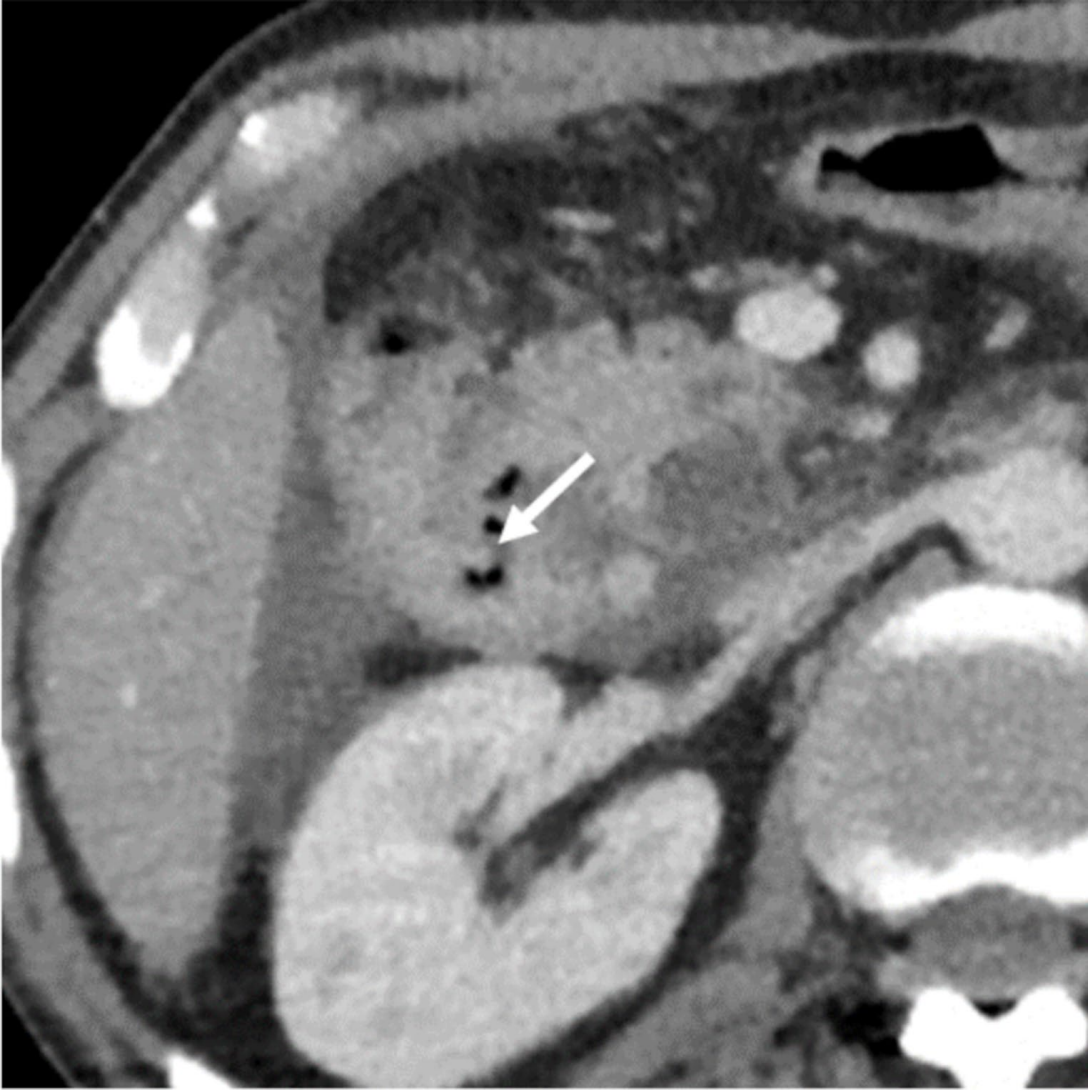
Contrast-enhanced abdominal CT. Wall defect was visible on the descending part of the duodenum (arrow) with extraluminal air and fluid in the pancreaticoduodenal recess and surrounding right liver lobe in keeping with the diagnosis of duodenal perforation
